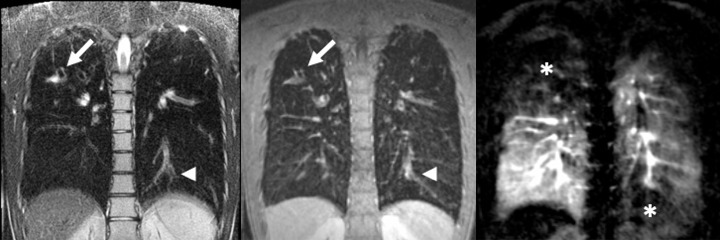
MRI of bronchiectasis and perfusion deficits in a 15-year-old patient with severe CF. *T*
